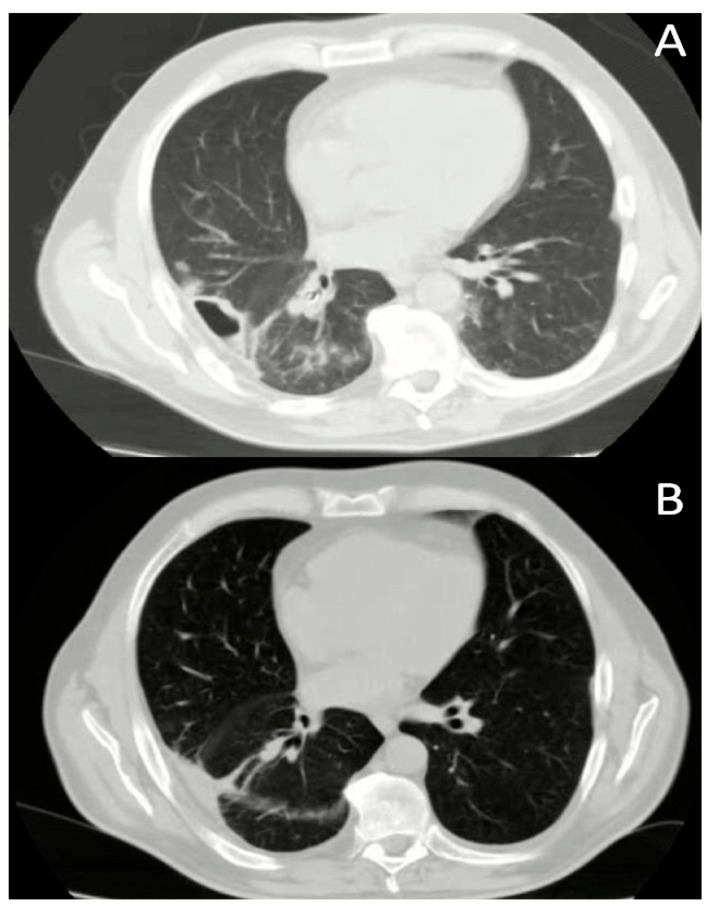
Chest computed tomography scan before (A) and after treatment (B).
